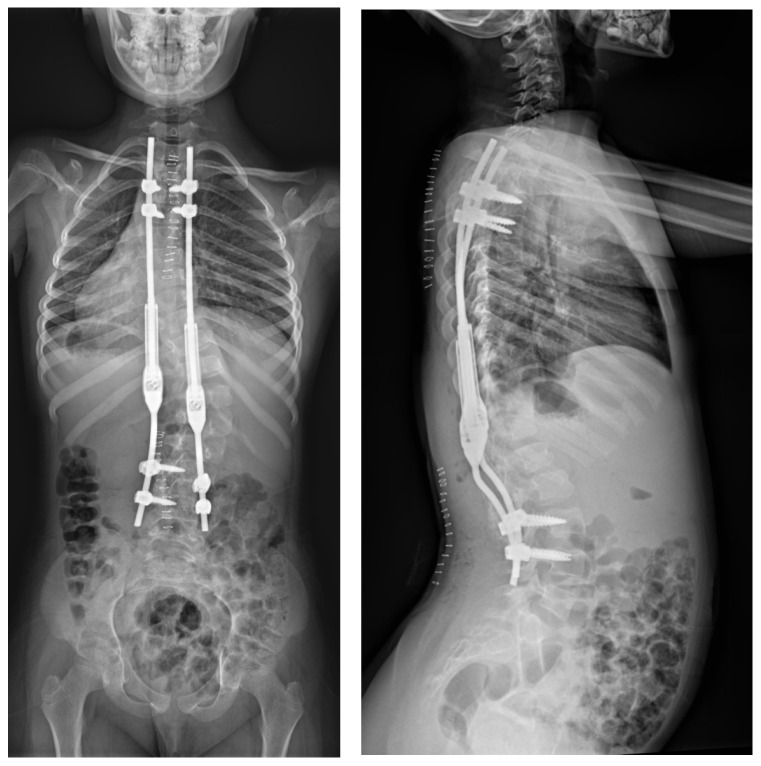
Standing postoperative X-rays (LAT and AP).
